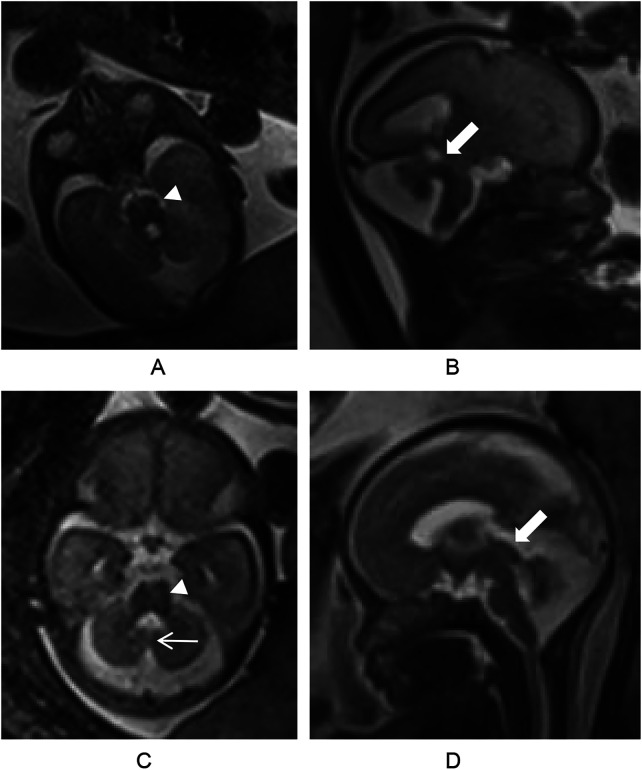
MRI of the fetuses. (A) Figure Axial half-Fourier acquisition single-shot turbo spin echo (HASTE) image showing the molar tooth sign of the first fetus(arrowhead). (B) Sagittal HASTE image showing a widened posterior fossa, cerebellar vermis dysplasia, and thickened and elongated superior cerebellar peduncles(thick arrow). The enlarged fourth ventricle communicated with the cisterna magna. (C) Axial HASTE image showing the molar tooth sign(arrowhead) and enlarged fourth ventricle of the second fetus,as well as the "cleft sign" (thin arrow). (D) Sagittal HASTE image showing cerebellar vermis dysplasia, and thickened, elongated superior cerebellar peduncles(thick arrow).
